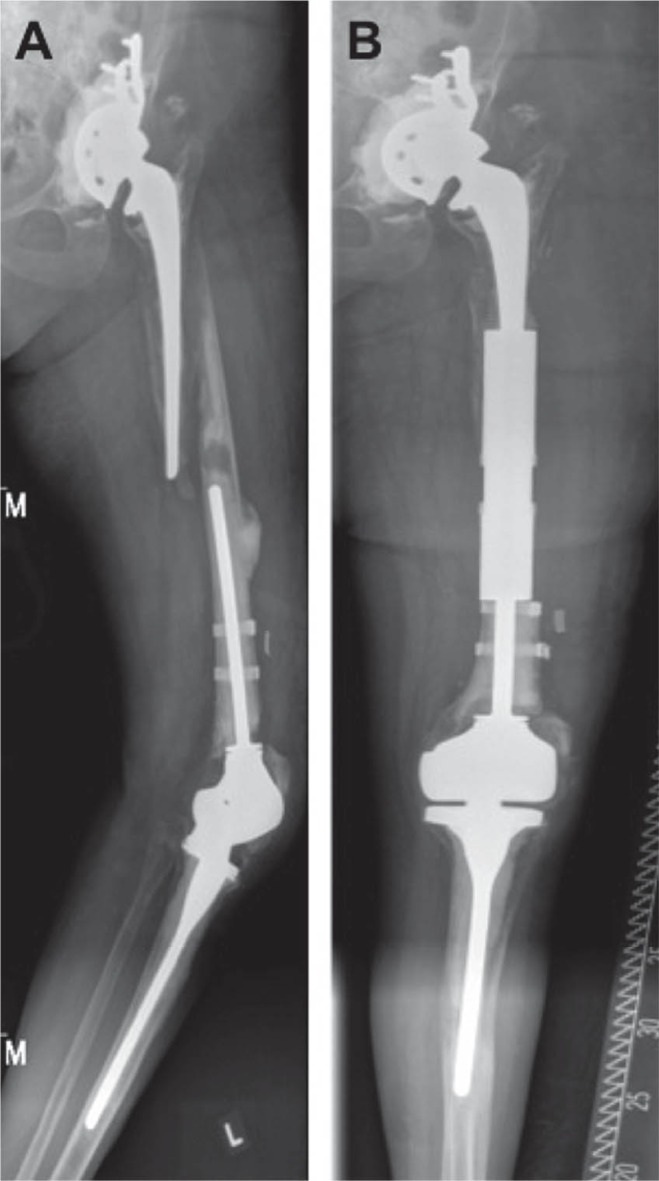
A. Interprosthetic femoral fracture in a 78-year-old woman. B. Follow-up radiograph after 1.5 years. At this stage the patient was mobile with a walker and free of pain (HSS 55).
